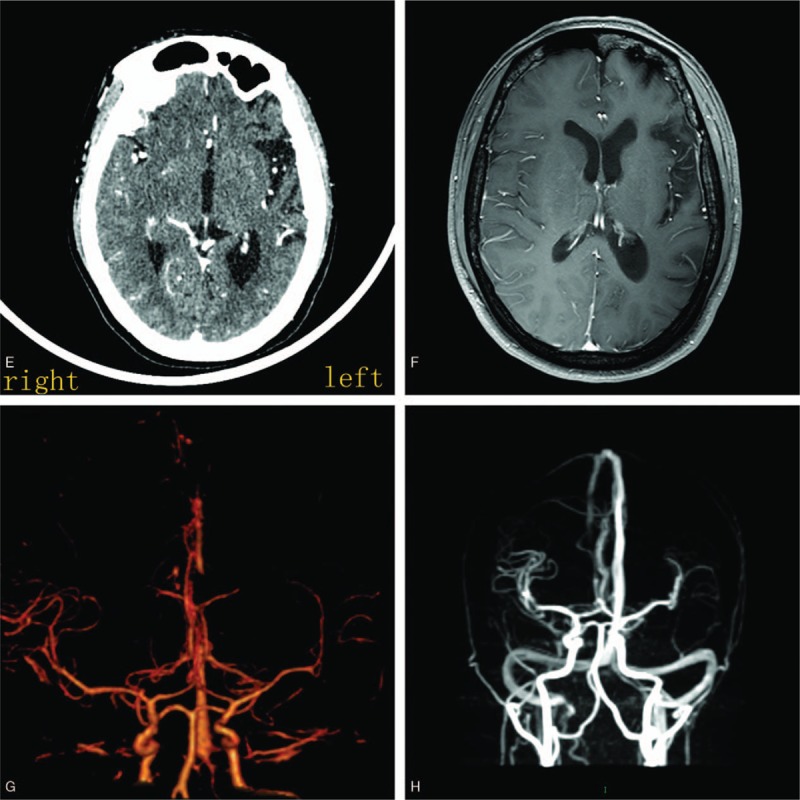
(A) Enhanced computed tomography examination showing fewer blood vessels in left cerebral hemisphere. (B) Enhanced MRI showing fewer blood vessels in left cerebral hemisphere. (C) computed tomography angiography showing fewer blood branches in left the middle cerebral artery. (D) Magnetic resonance venography showing fewer blood branches in left middle cerebral vein.
